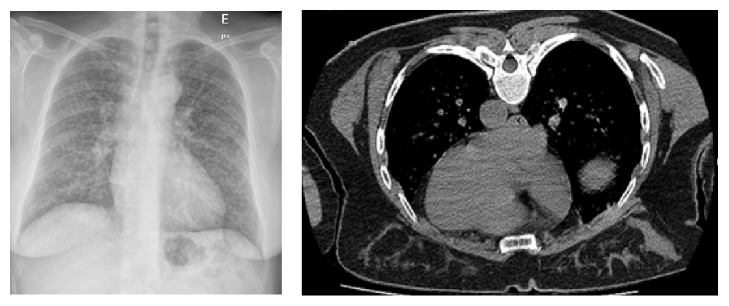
Chest radiography and CT images of patient 1.
